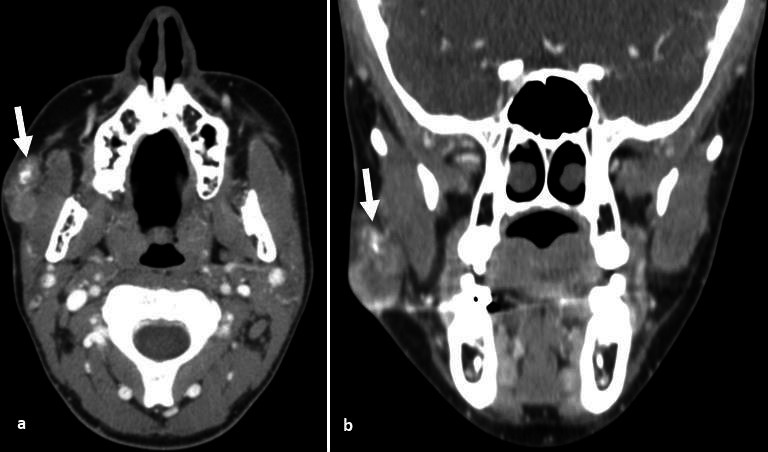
Pilomatricoma. Axial and coronal CT post-contrast demonstrates a lobulated, well-circumscribed buccal cutis and subcutis mass, superficial to the right masseter with linear foci of calcification (arrows) and mild enhancement.
